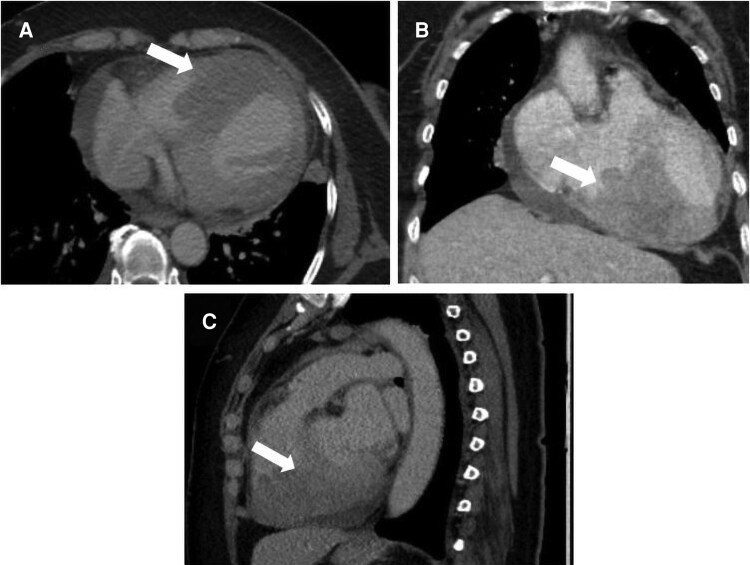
Cardiac computed tomography with contrast showing an enhancing infiltrative soft tissue cardiac mass extending to the right ventricle with involvement of the interventricular septum and the left ventricular inferior walls (*A*, *B*, *C*, white arrows).
